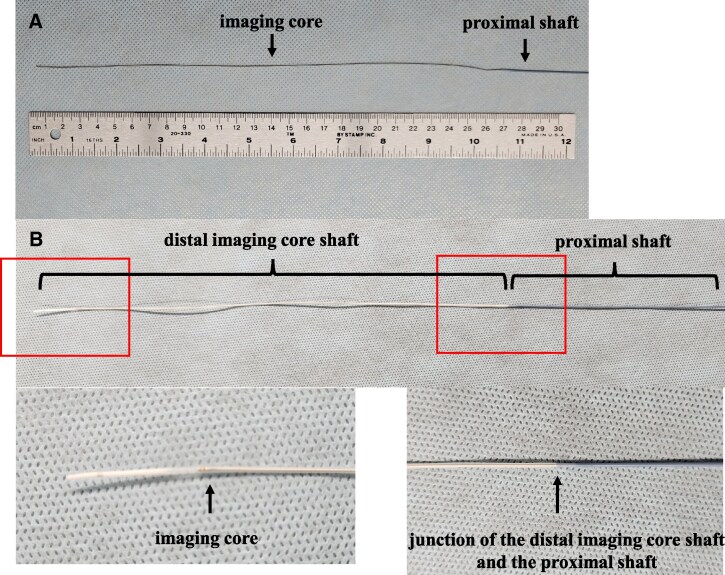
The structure of the intravascular ultrasound catheter. (*A*) The top part of the broken intravascular ultrasound catheter. With a distal imaging core shaft loss, the imaging core is exposed to the outside of the shaft. (*B*) Upper: an appearance of a normal intravascular ultrasound catheter. Lower left: the tip of the imaging core in the distal imaging core shaft. Lower right: the junction of the distal and proximal core shafts.
